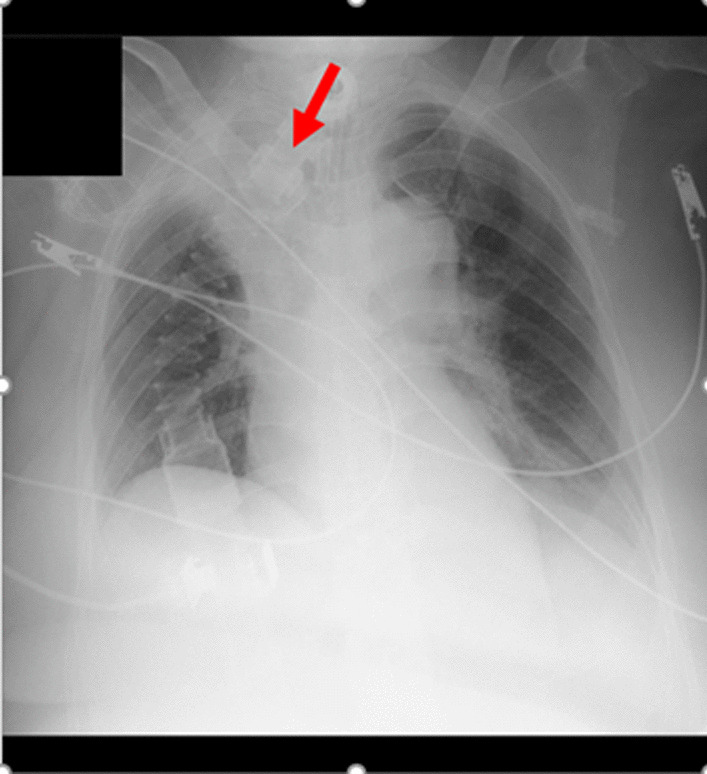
Chest radiograph. Red arrow indicates presence of atelectasis
